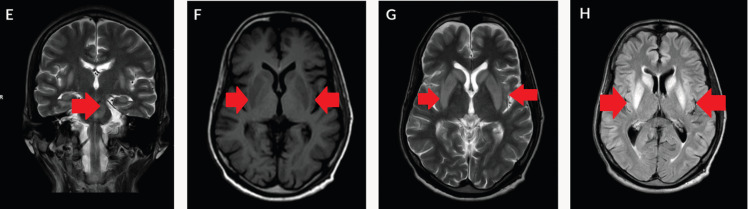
Evidence of altered signal intensity lesion in pons appearing hyperintense on coronal T2WI (E); in the bilateral caudate nucleus, lentiform nucleus, posterior limb of the internal capsule, and external capsule appearing hypointense on axial T1WI (F), hyperintense on T2WI/FLAIR (G, H) in the axial section FLAIR: fluid-attenuated inversion recovery
